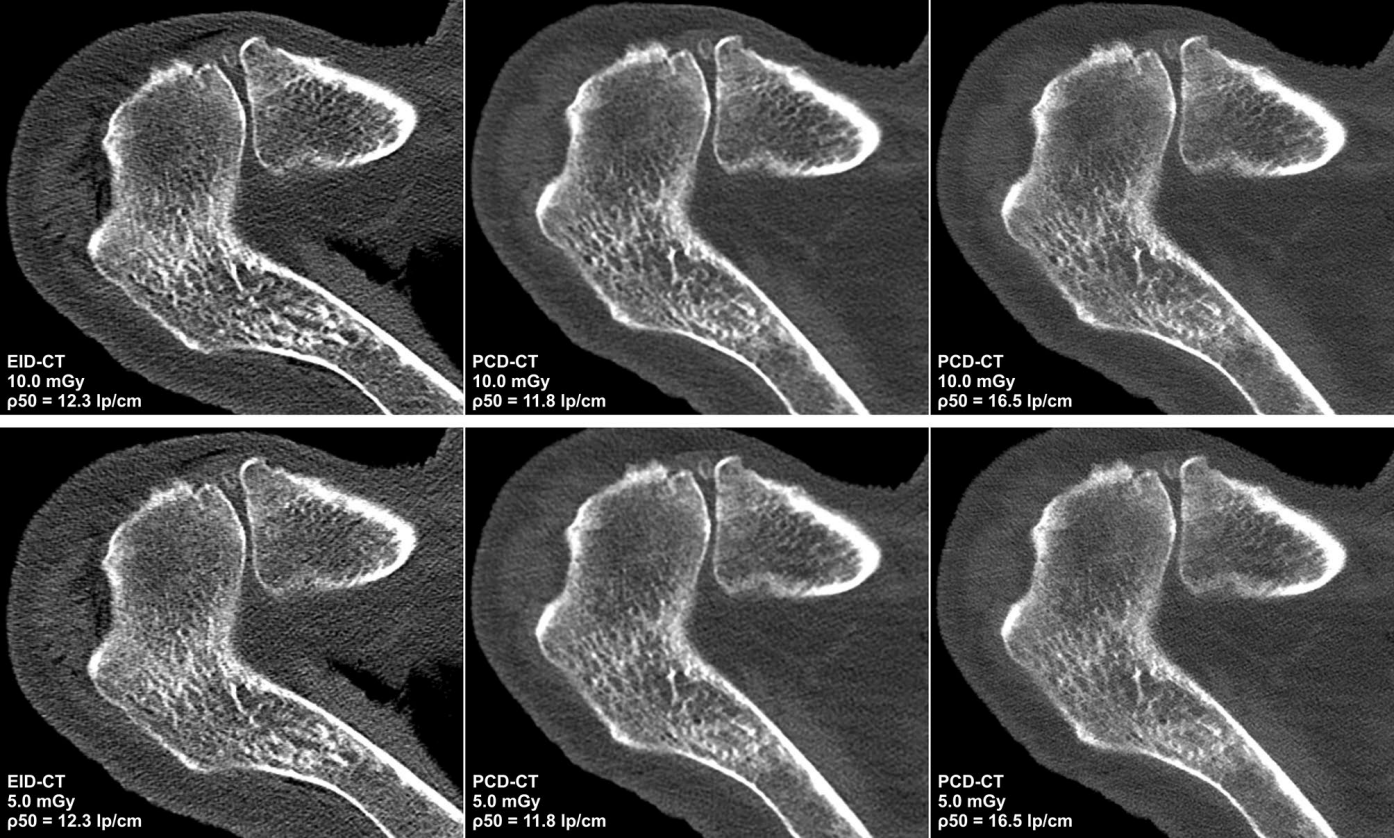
Representative axial CT slices at the level of the acromioclavicular joint demonstrate the image quality with all six combinations of dose protocol and detector technology. Increased image noise with lower dose in EID-CT limits assessability of cancellous bone structures. Upper row: Full-dose scan protocols (10.0 mGy): EID-CT, non-UHR-PCD-CT, UHR-PCD-CT. Lower row: Low-dose scan protocols (5.0 mGy): EID-CT, non-UHR-PCD-CT, UHR-PCD-CT.
